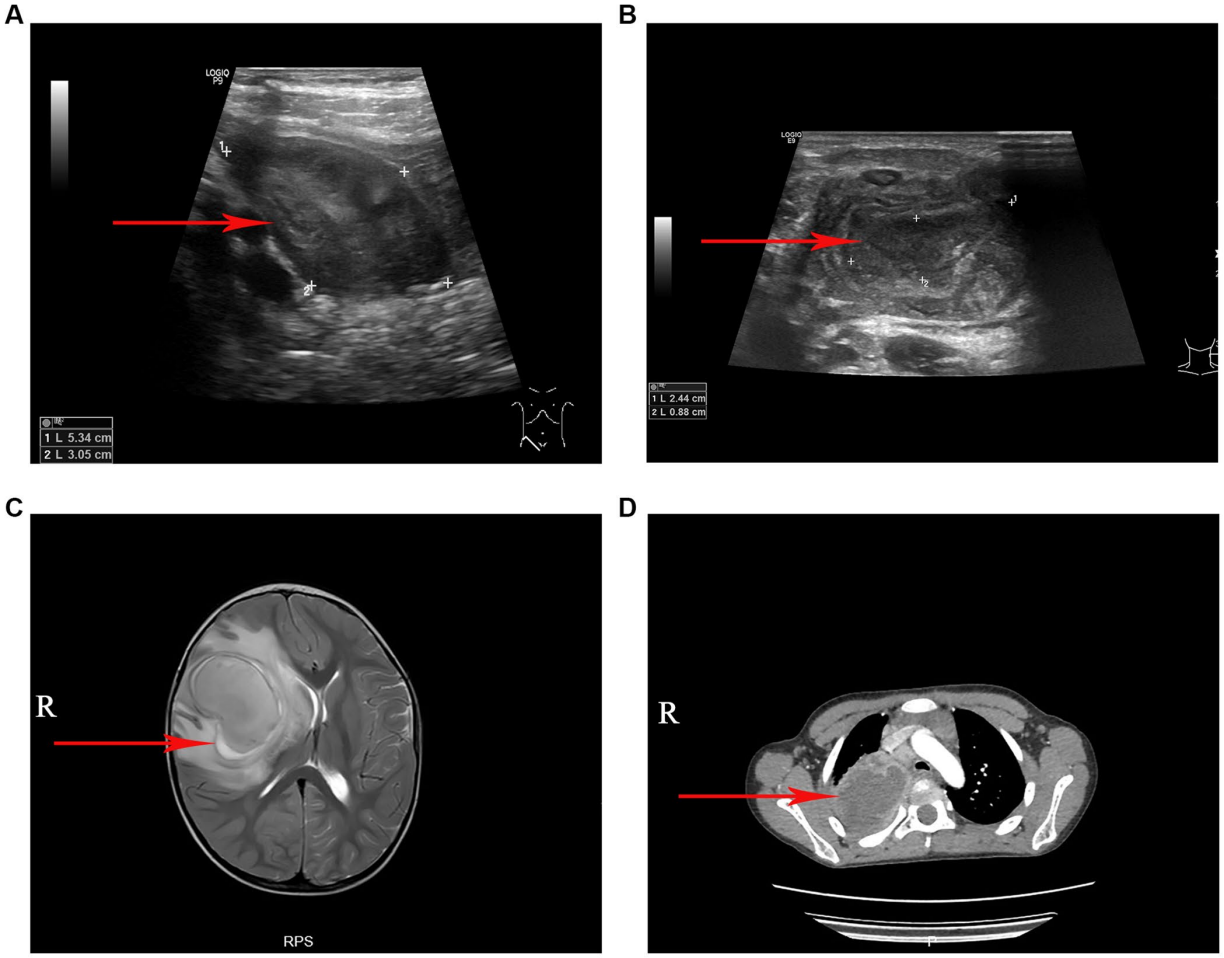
The imaging manifestations of *S. intermedius*. (A) Ultrasound examination revealed a local abscess formation around the appendix. (B) Ultrasonography revealed a cervical lymph node abscess formation. (C) Magnetic resonance imaging (T2-weighted) showed a large cerebral abscess in the right cerebral hemisphere. (D) Chest computed tomography showed a pulmonary abscess in the upper lobe of the right lung with surrounding inflammatory changes.
